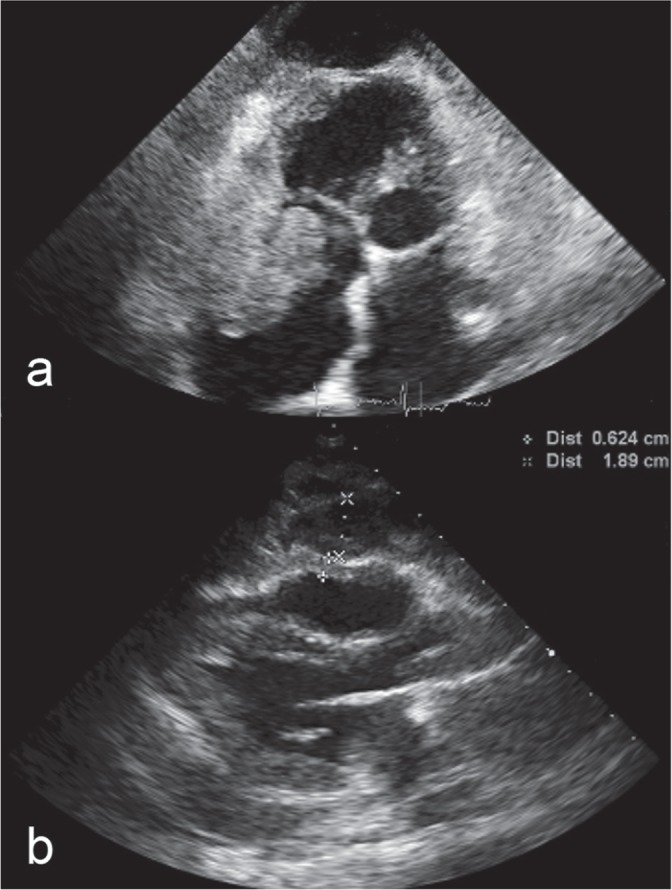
Echocardiography examination revealing (a) tumorous mass protruding into the right atrium and (b) soft tissue filling the pericardial sac around both ventricles.
